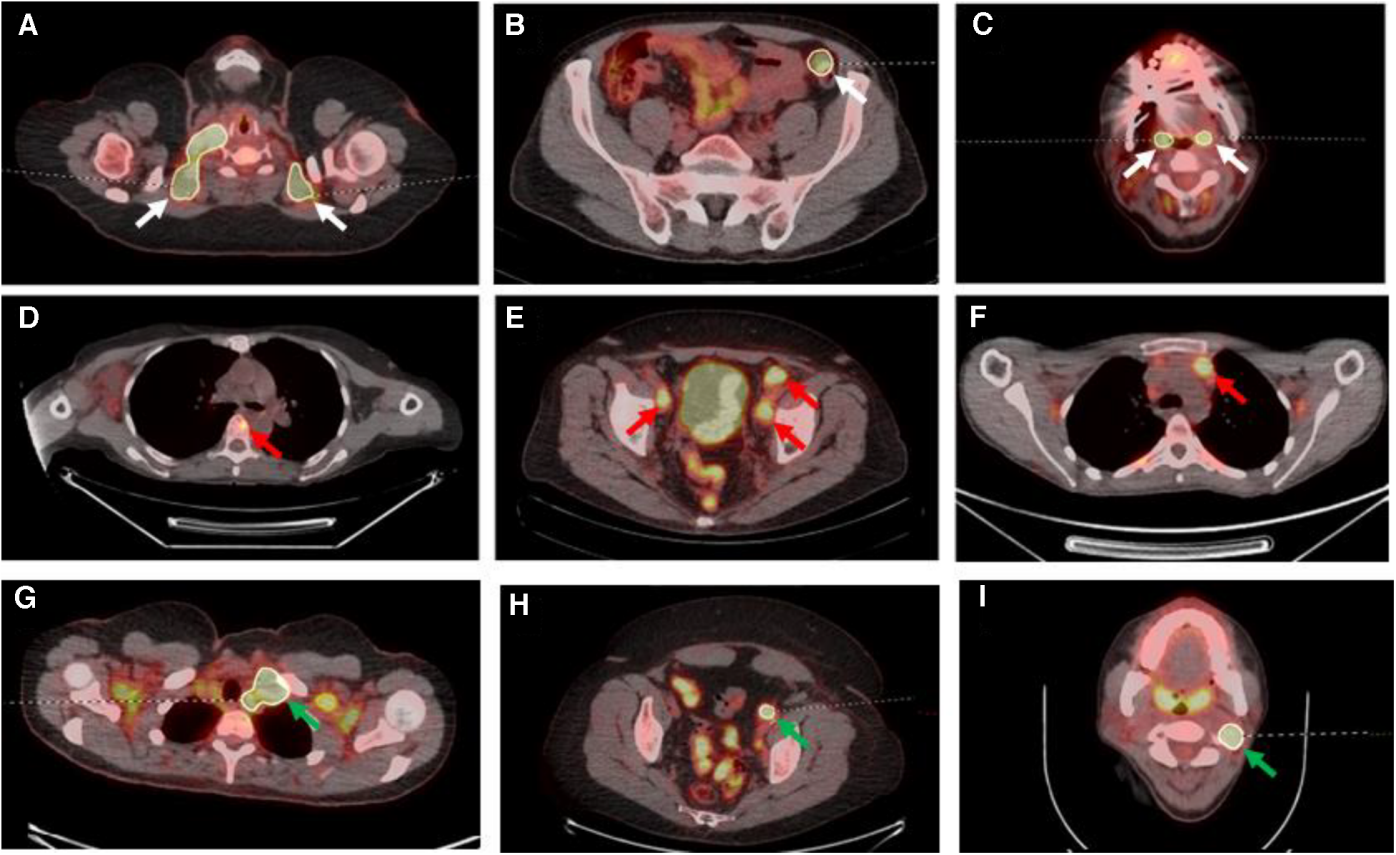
Select axial fused PET/CT slices depicted false positive segmentations (A–C), false negative segmentations (D–F) and gold standard (G–I). (A) Segmentations of brown fat; (B) the incorrect segmentation of the descending colon in a patient on metformin; (C) the segmentation of the palatine tonsils; (D) the missing segmentation of osseous involvement of a vertebral body (red arrow); (E) the missing segmentation of pelvic sidewall nodal disease (red arrows); (F) the missing segmentation of anterior mediastinal nodal disease (red arrow); (G) correct segmentation of left level IV lymph node (green arrow) on a background of brown fat uptake; (H) correct left external iliac lymph node (green arrow) segmentation on a background of metformin bowel uptake; (I) correct left level II lymph node segmentation (green arrow) on a background of physiological palatine tonsil uptake.
